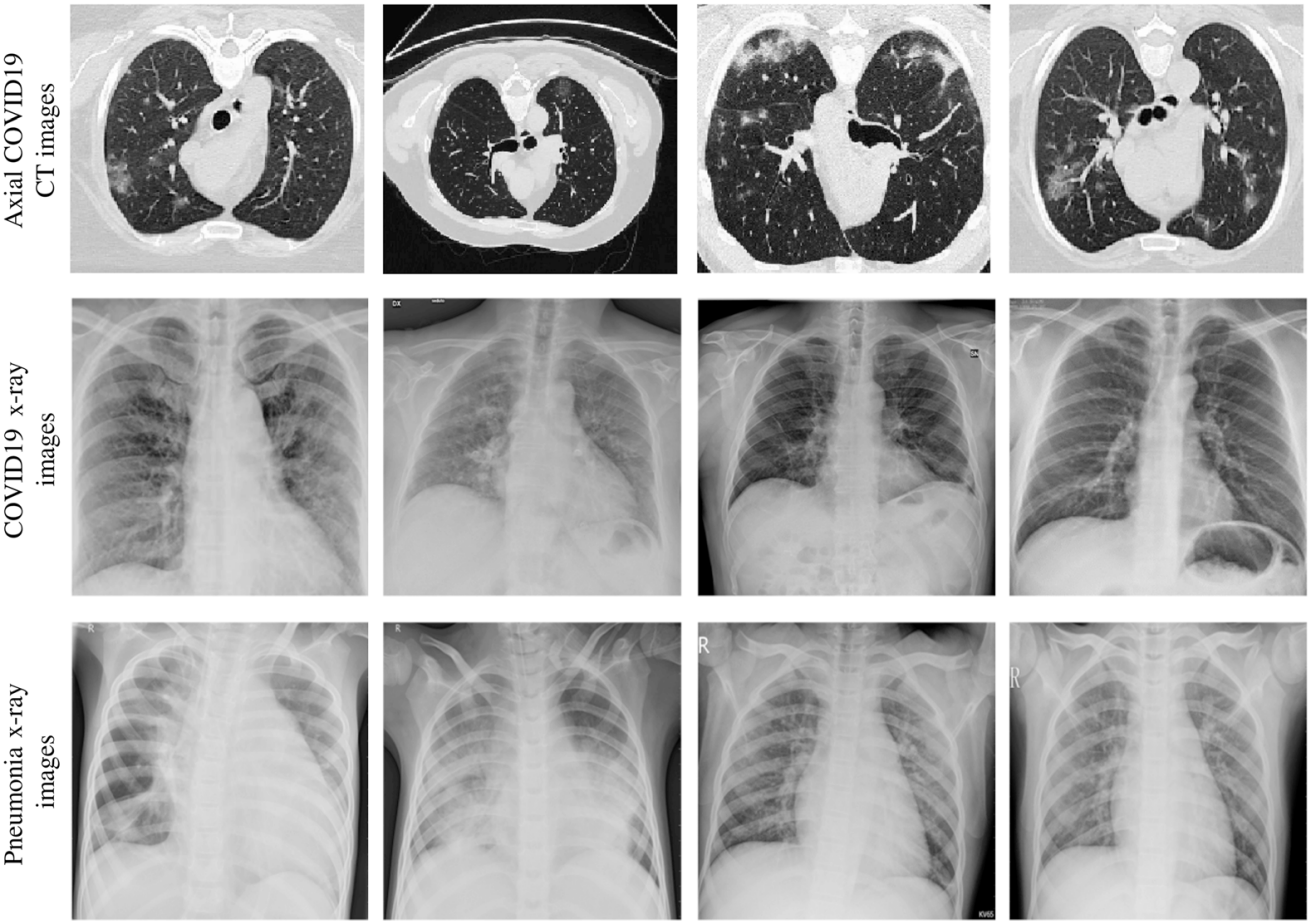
Examples of COVID-19 in CT and x-ray images. First row: axial COVID-19 CT images with lesions in different positions and sizes. Second row: COVID-19 x-ray images. Third row: pneumonia x-ray images.
